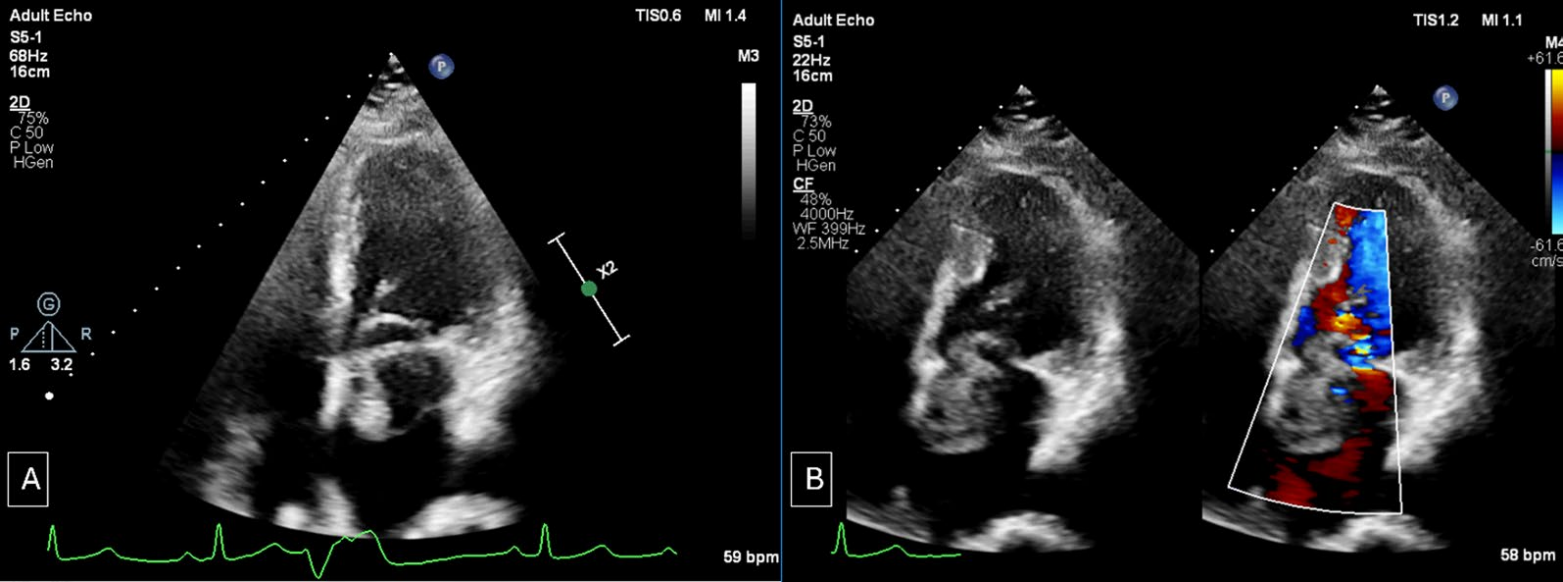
Apical four-chamber view demonstrating complex solid cystic mass lesion in left atrium protruding across the mitral annulus (A) causing dynamic transmitral inflow stenosis during ventricular diastole (B)
